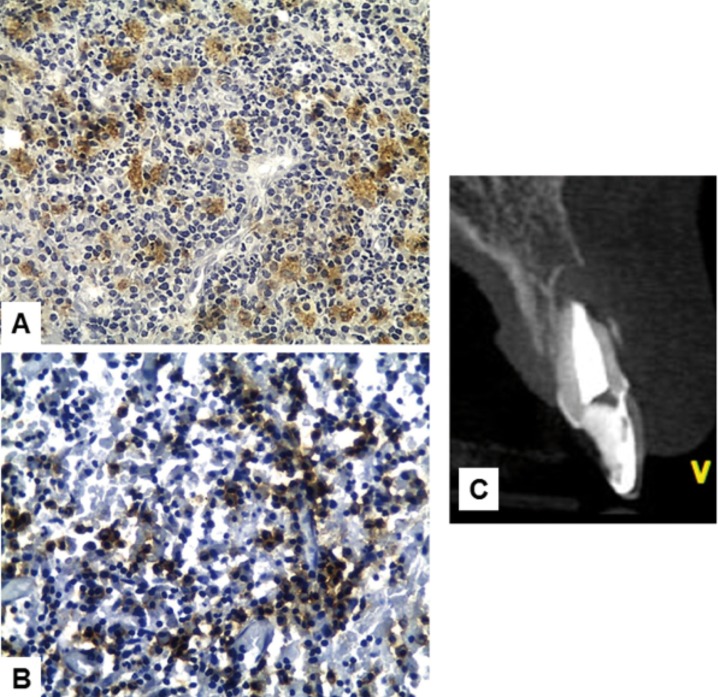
Periapical granuloma. A: CD68+ macrophages. B: CD138+ plasma cells (immunoperoxidase, original magnification, x400). C: tomographic image.
